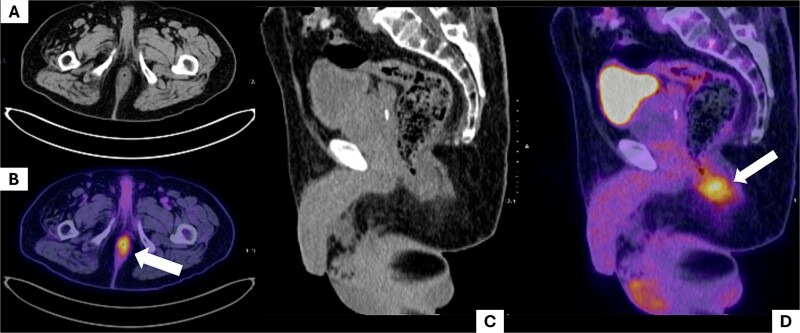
Positron emission tomography (PET-CT) scan. The images show the region corresponding to the anal canal. A and B show the affected anatomical region in axial slices. (A) Shows the anal canal with slight asymmetrical thickening predominantly on the left side, with no suspicious adenopathies; (B) shows uptake corresponding to the increase in local metabolism secondary to the tumor lesion (white arrow). C and D show sagittal slices of the pelvic region, where uptake is observed at the level of the anal canal (white arrow).
